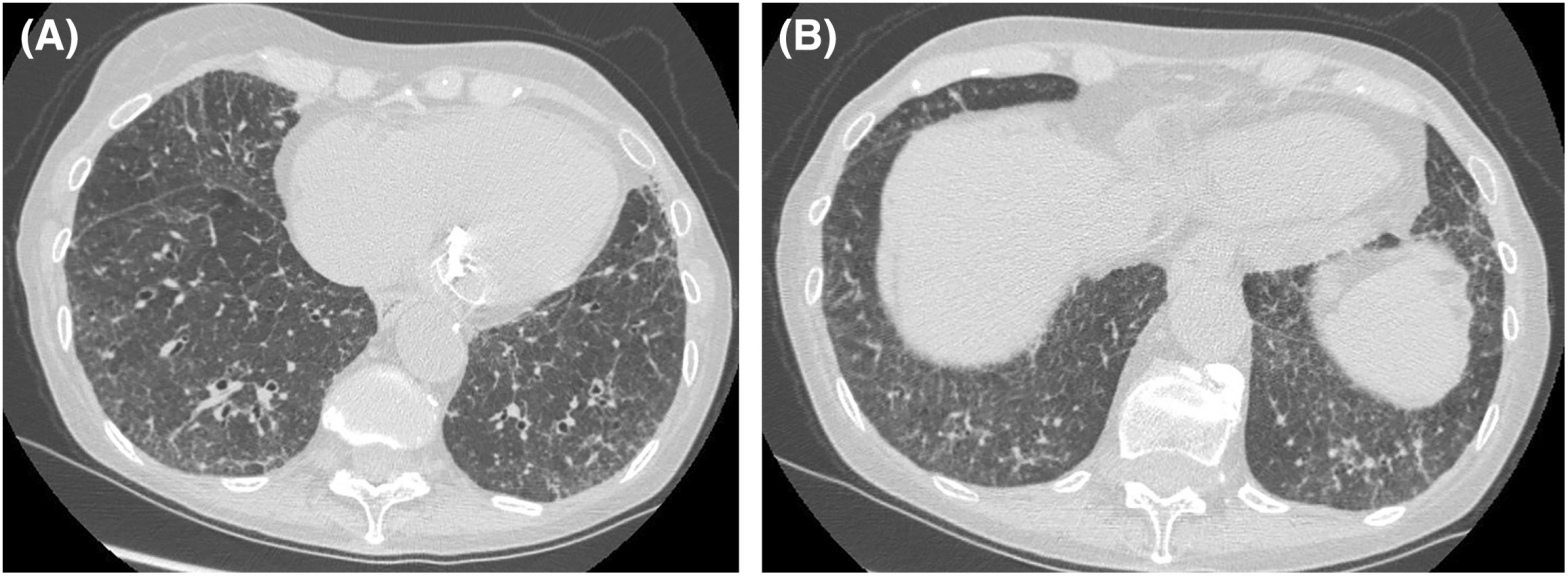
(a) Lung HRCT, November 2023: Reduction of lung abnormalities, still predominant at the periphery of lung bases. (b) Ground glass opacities are the main alterations.
